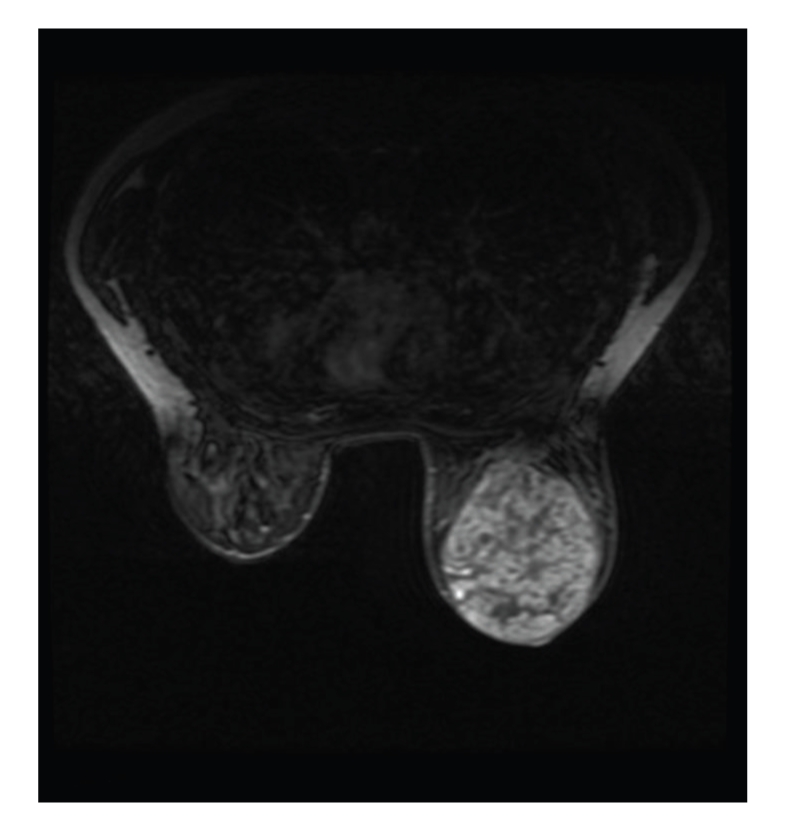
MRI showing the 10 cm well-circumscribed rounded mass lesion which demonstrated a benign enhancement pattern after intravenous Gadolinium.
